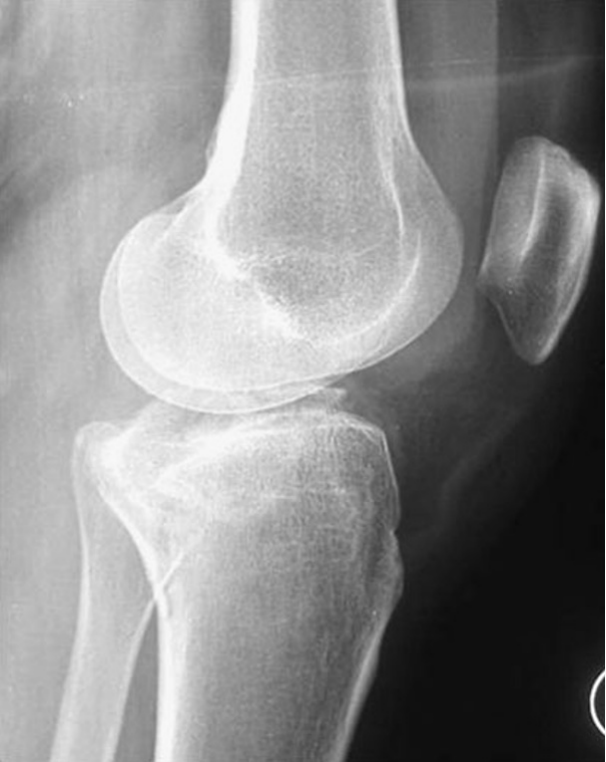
Schatzker type V fracture, pre-op X-rays (LL)
